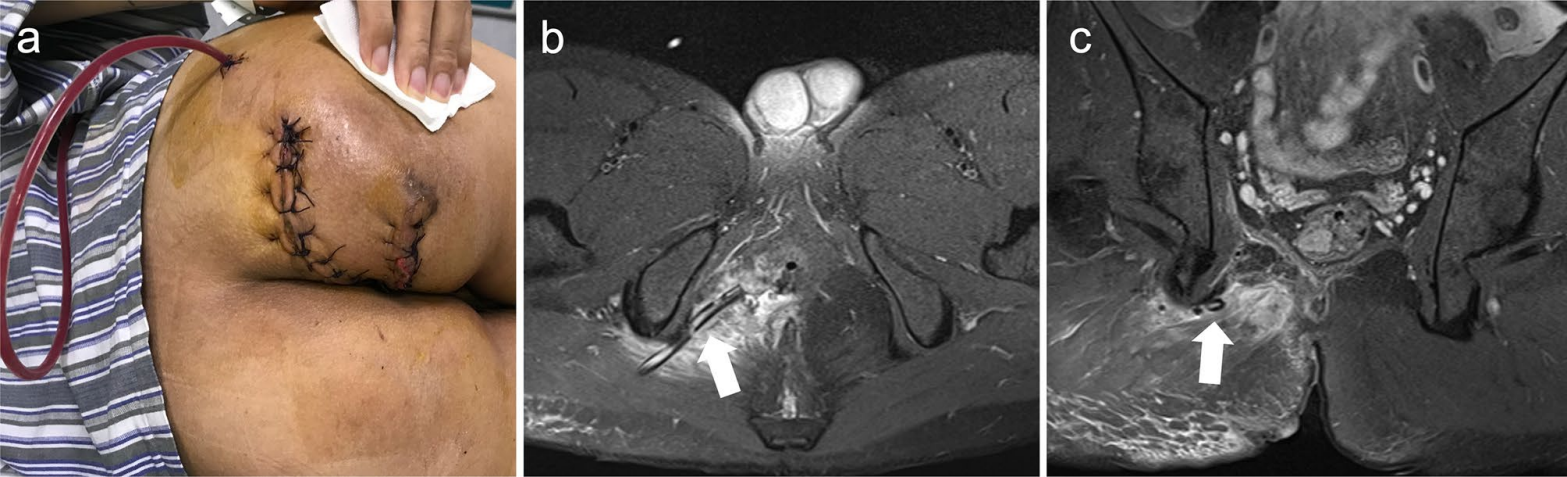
After reexamination of MRI on postoperative day 8, it was found that there was no obvious effusion, and then the drainage tube was removed. b–c MRI on postoperative day 8 (white arrow: drainage tube)
